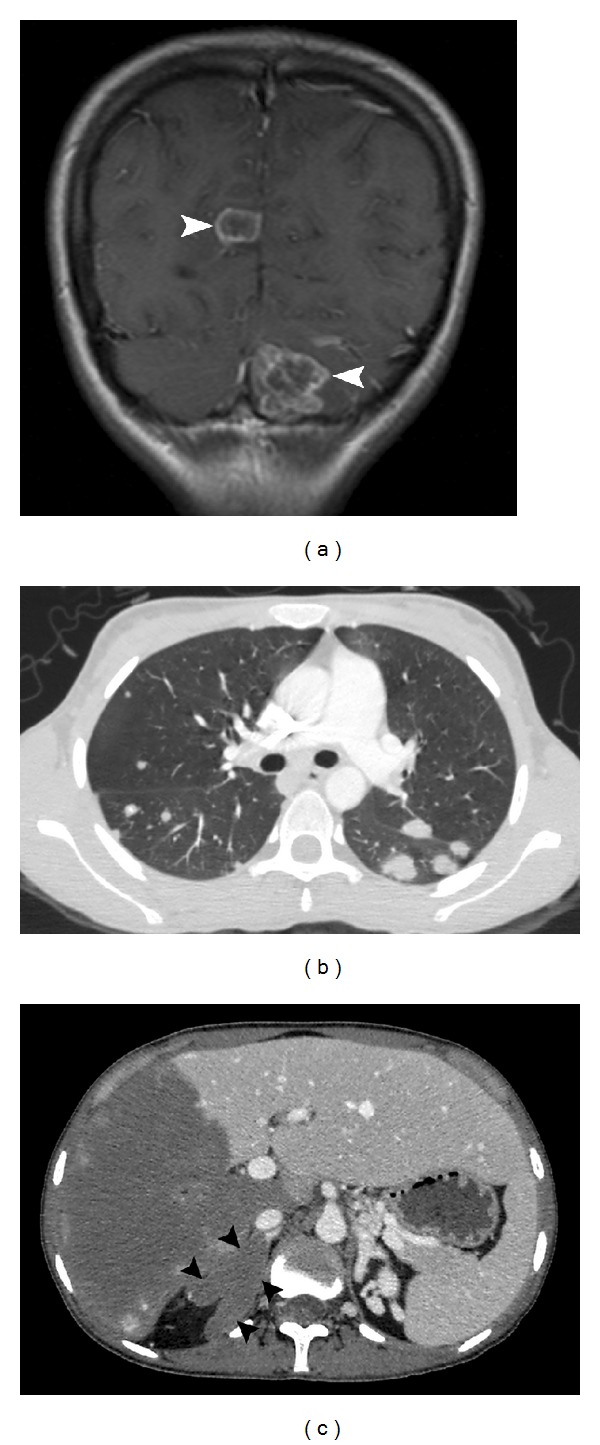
Postcontrast coronal T1-weighted spin echo (SE) image (a) showing irregular peripheral enhancement at the lesions (arrowheads). Chest CT image (b) demonstrates bilateral pulmonary involvement of alveolar hydatid disease. Diffuse right adrenal gland enlargement (arrowheads) is present on contrasted CT (c) compared to the left gland.
